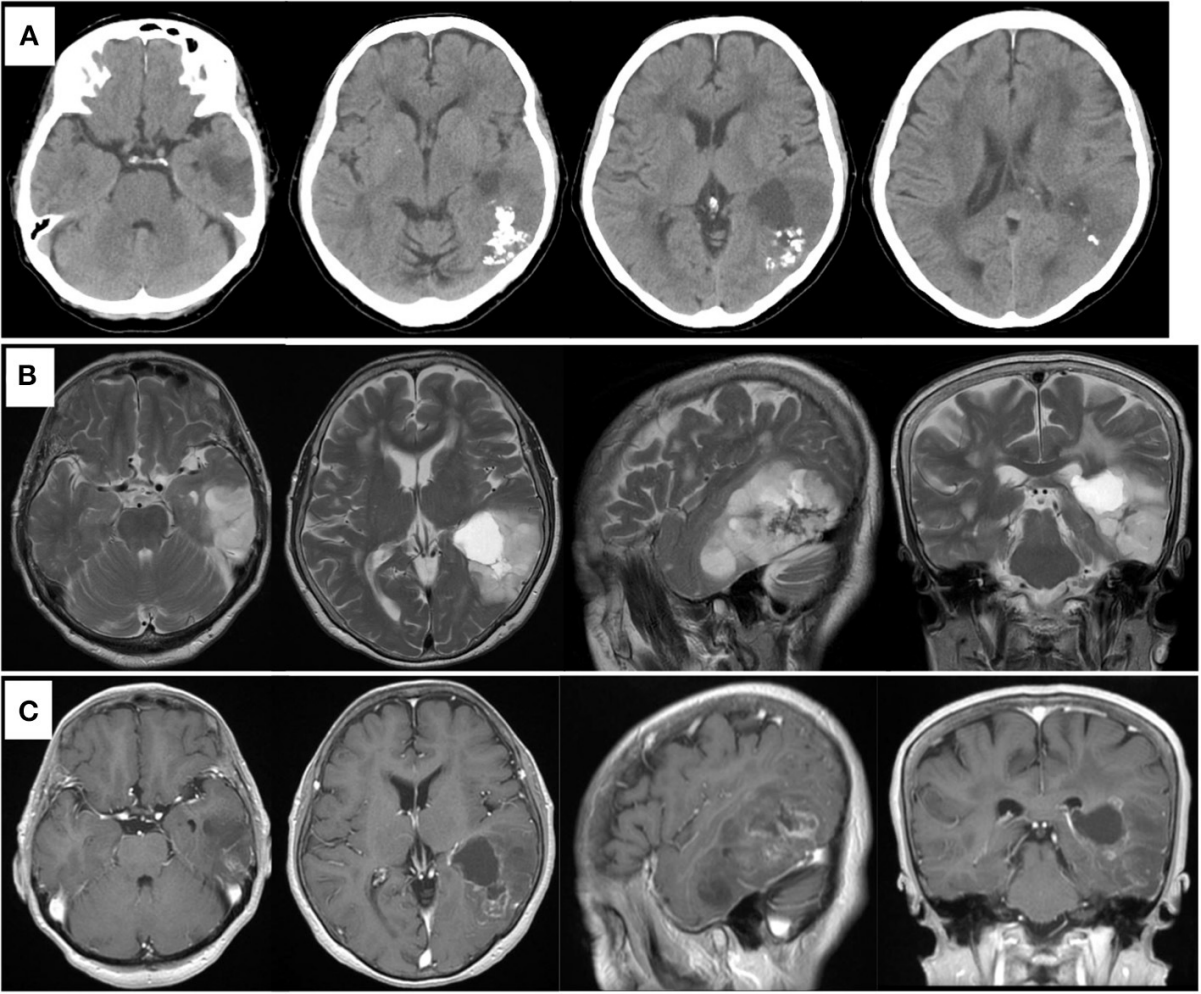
Preoperative computed tomography showing calcified and cystic lesions in the left temporal lobe (A). MR images showing high intensity in the left temporal lobe on T2-weighted image (B) and heterogeneous enhanced lesion on gadolinium-enhanced T1- weighted image (C).
